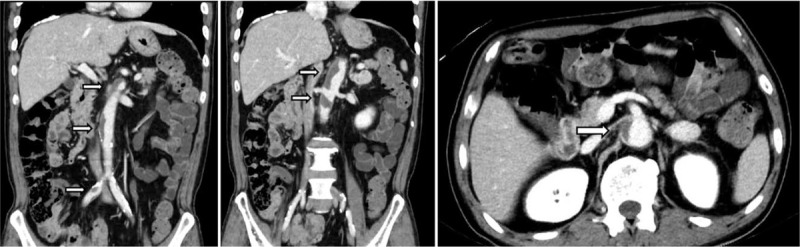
Contrast-enhanced CT on day 21: thrombotic deposits (arrows) were identified in the abdominal aorta and common iliac artery without any occlusive lesion or progression of cancer. CT = computed tomography.
